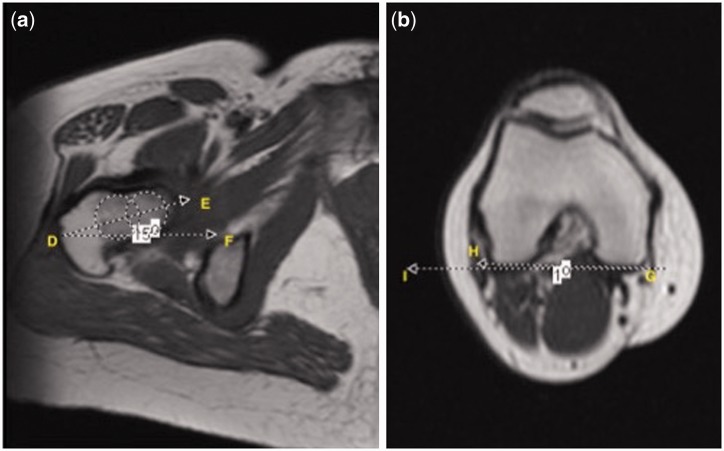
Axial T1-weighted MR image shows the representation of the: (a) Angle DEF representing the Femoral Neck axis. Two centroids were positioned through the femoral neck shaft. FNa was formed by the angle of the line passing through the middle of the both centroids relative to the horizontal line; (b) posterior condylar axis of the knee as the angle IGH.
